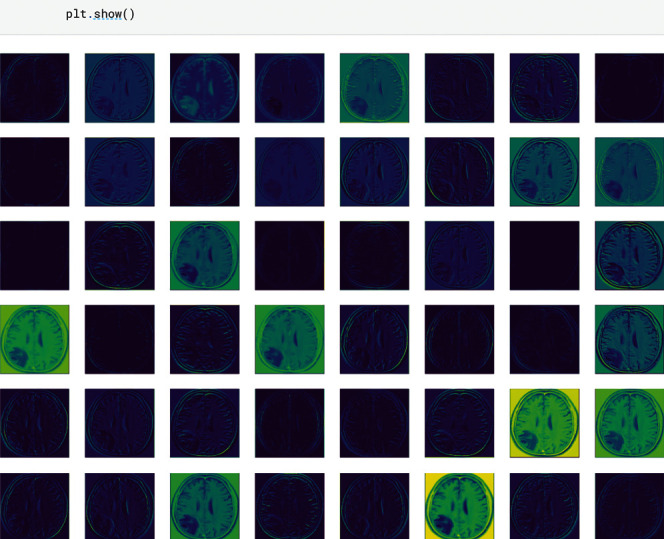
VGG-16 classification process loaded with MRI image data [22].
